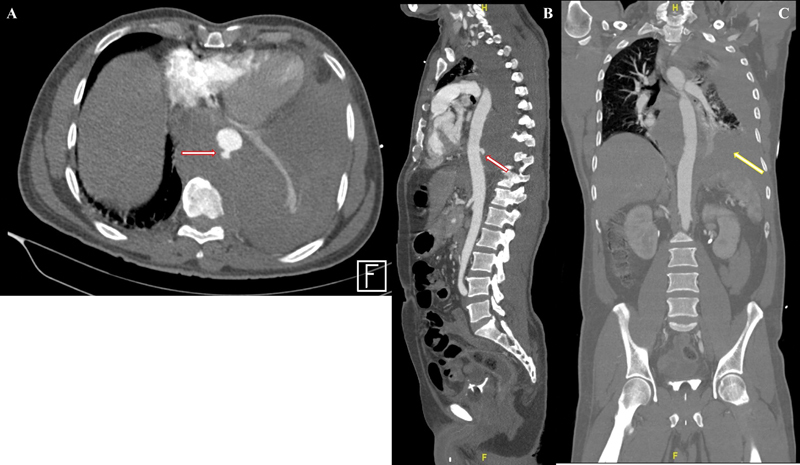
Computed tomography (CT) aortogram of the thoracic aorta
